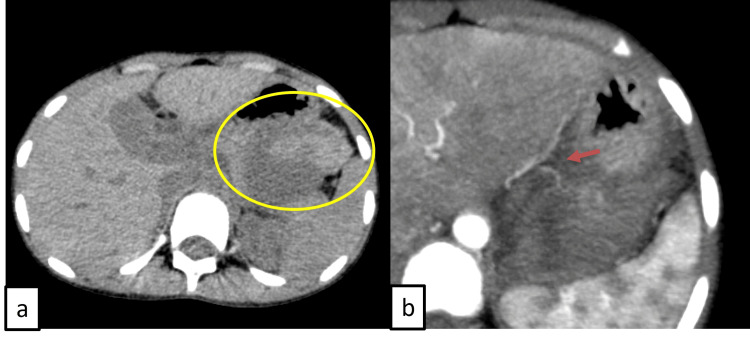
Preoperative computed tomography of the abdomen showing intralesional bleed a: Hyperdense area of hemorrhages (axial view). b: Left gastric artery feeder vessel.
